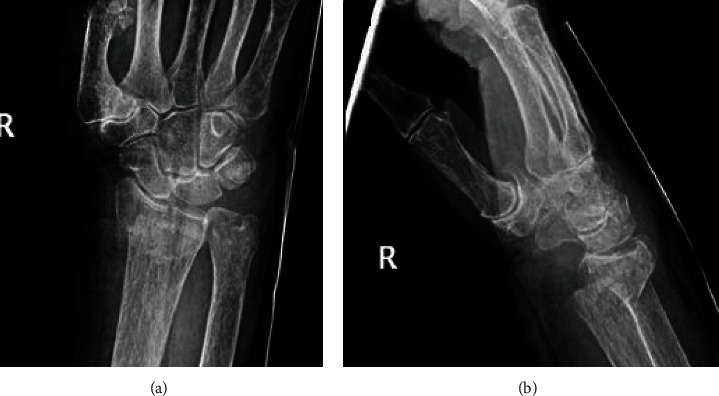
Plain radiographs of the right wrist in the (a) AP and (b) lateral views showing a dorsally displaced distal radius fracture with intra-articular extension.
